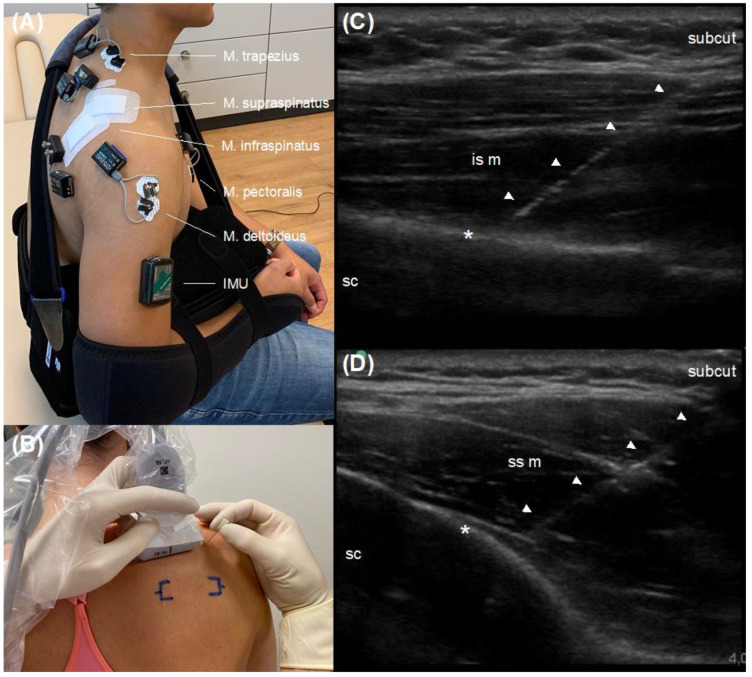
Placement of the electromyography electrodes on the investigated shoulder muscles. The placement of the inertial measurement unit is also shown. Note: (A) placement of electrodes and the inertial measurement unit (IMU); (B) sonography-guided application of the fine-wire electrodes; (C) positioning of electrode in the infraspinatus muscle; (D) positioning of electrode in the supraspinatus muscle. Abbreviations: subcut = subcutaneous fat tissue; is m = infraspinatus muscle; sc = scapula; ss m = supraspinatus muscle; asterisk = cortical interface; arrows = intramuscular needle position.
